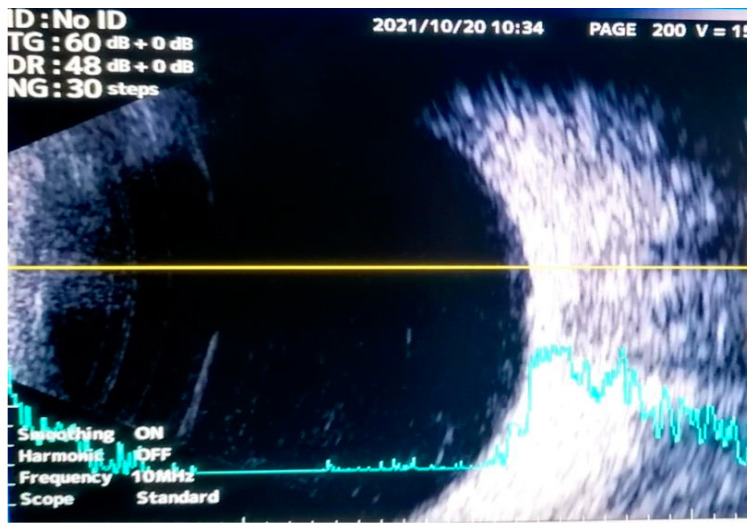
Standardized B-scan ultrasonography of the left eye did not reveal the presence of any intraocular mass. Computed tomography (CT) of the brain and orbit showed a front-ethmoid tissue mass with bone destruction and regional invasion (Figure 3).
